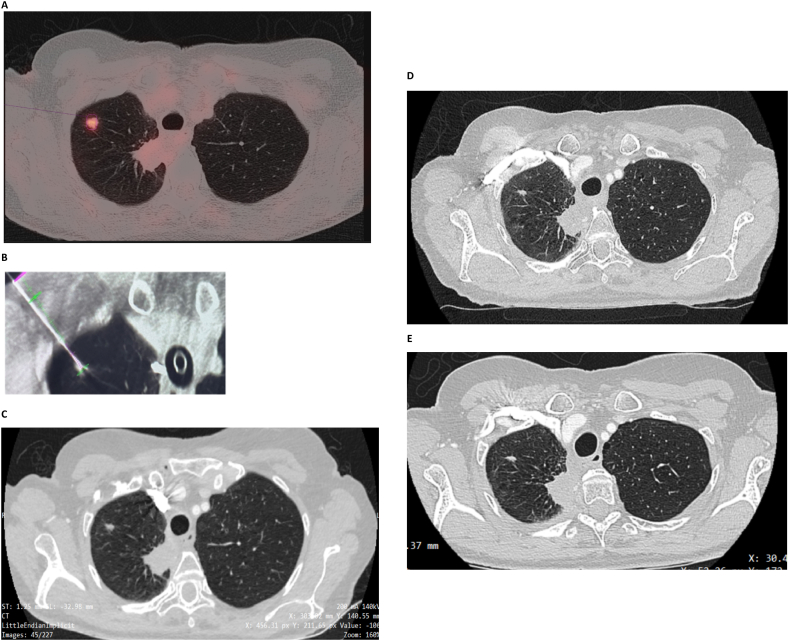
A) Axial PET-CT scan images of chest from March 2022 showing new 9 mm RUL and stable right paramediastinal disease; B) Percutaneous needle prior to delivery of PEF from June 2022; Follow-up images performed at 3-months (C) and 6-months (D), and 12 months (E).
